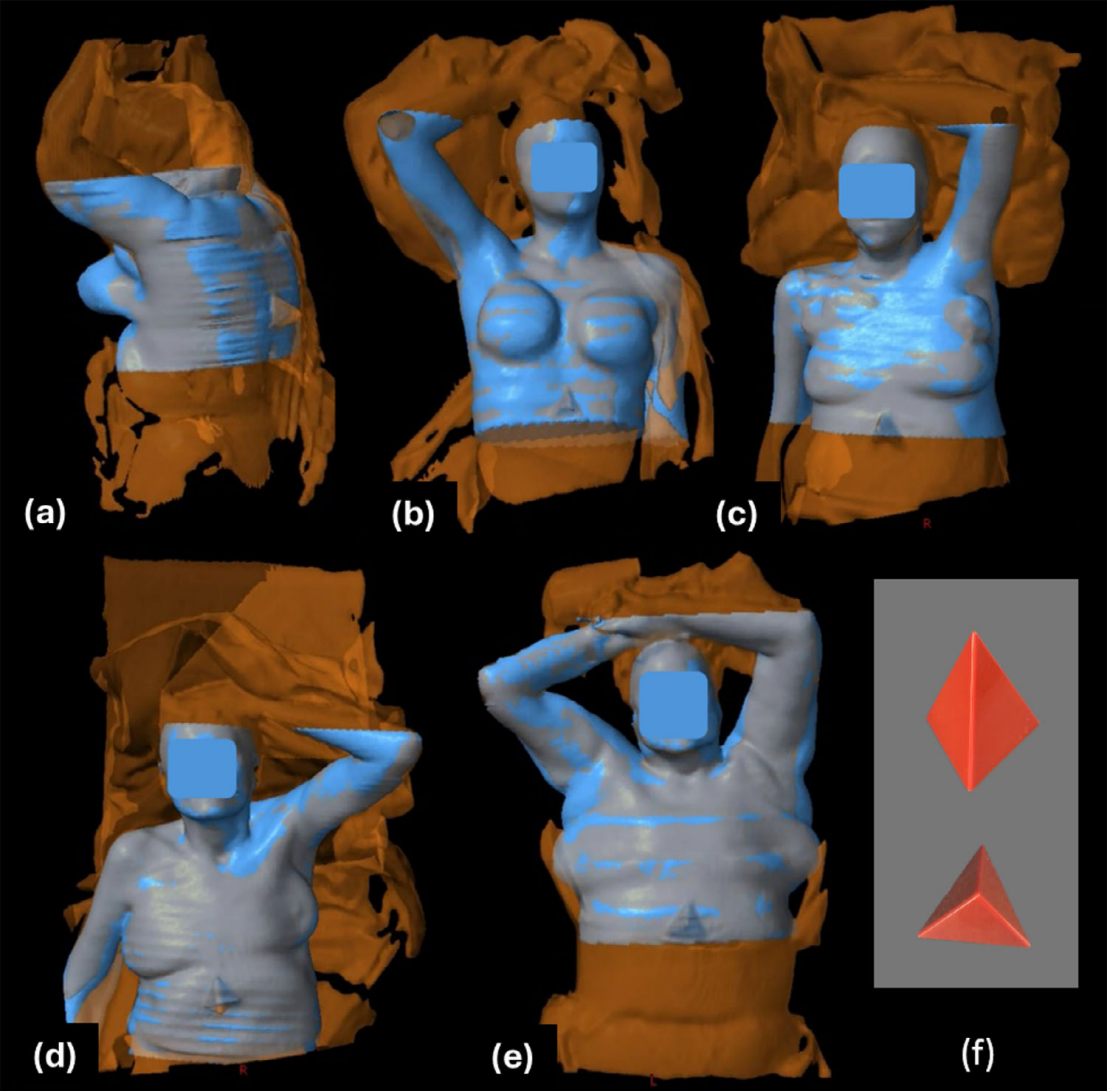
Overlay of CT image surface (blue) and 3D scan surface (orange). (a) Patient 1, (b) Patient 2, (c) Patient 3, (d) Patient 4, (e) Patient 5, (f) plastic pyramid patient marker. The 3D scan surface shows noticeably more information, including clipped arms and the patient immobilization.
